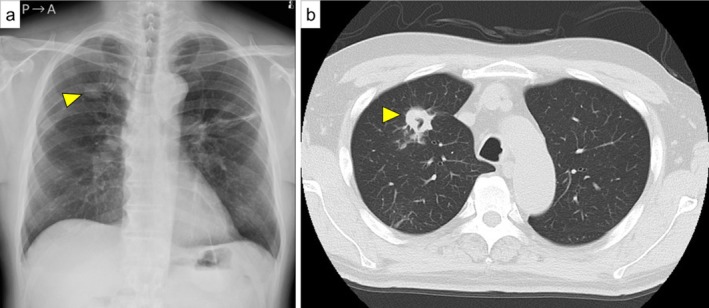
Chest imaging findings. (a) X‐ray showing a mass in the right upper lung field (arrowhead). (b) CT demonstrating a cavitary lesion (arrowhead).
